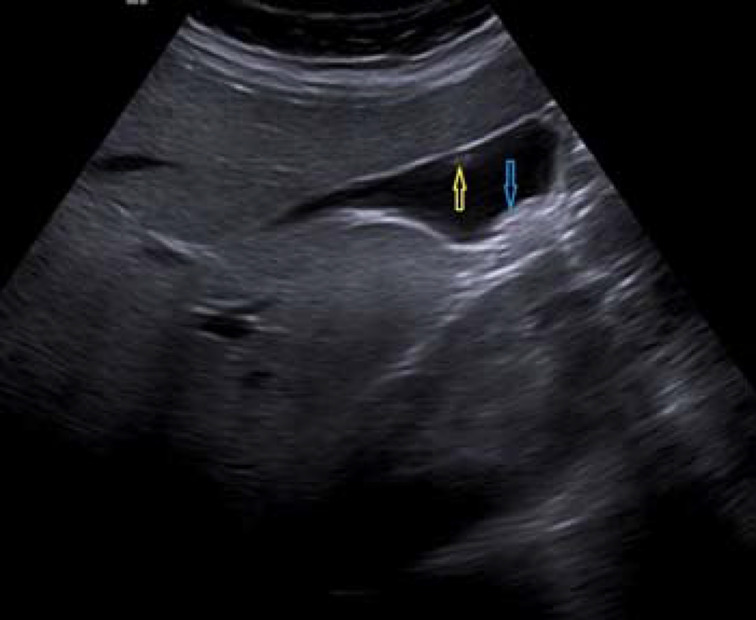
Ultrasound image showing gallbladder polyp (yellow arrow) and stones (blue arrow)
